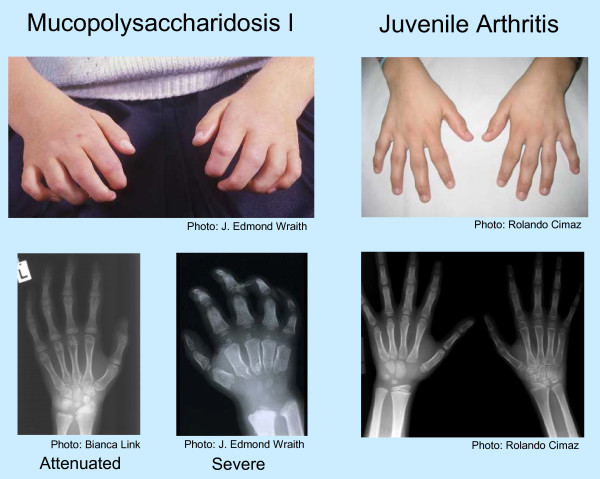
Physical and Radiographic Appearance of Hands in MPS I versus Juvenile Idiopathic Arthritis. The images on the left depict typical findings in MPS I - the curled "claw hand," abnormal metacarpal bones, proximal widening of phalanges, and the V-shaped deformity of the distal ulna and radius, particularly evident in severe MPS I. In contrast, the images on the right of a child with juvenile arthritis show the typical joint swelling and erosive bone lesions. Photos courtesy of J. Edmond Wraith, Rolando Cimaz, and Bianca Link.
